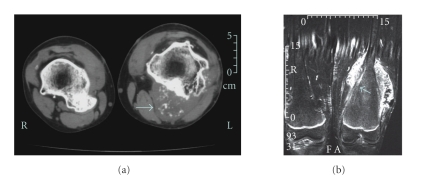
(a) CT scan includes both distal femurs prior to excision of osteochondromas. Contrast the benign appearance of the osteochondroma on the patient's right femur to the thickened cartilage cap with stippling on the left (arrow). (b)  MRI includes the left distal femur after resection of the benign posteromedial osteochondroma and biopsy of the posterior chondrosarcoma, with the thickened cartilage cap and invasion of femoral cortex (arrow) evident.
